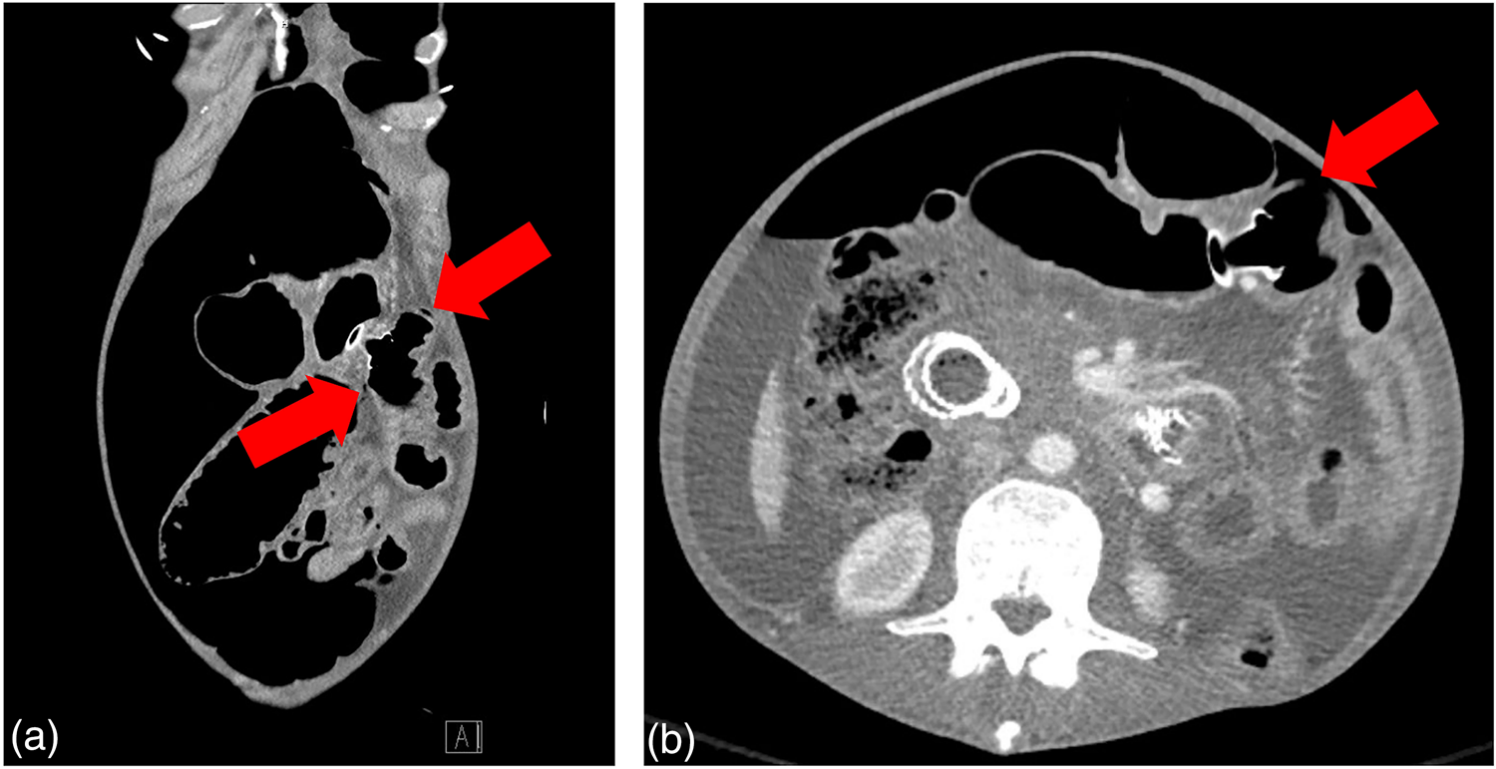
Computed tomography scan with flanking jejunal ulcerations indicated by arrows (a). The ulceration indicated on the patient's left side was perforated and this is better demonstrated on the cross‐sectional image indicated by an arrow (b).
